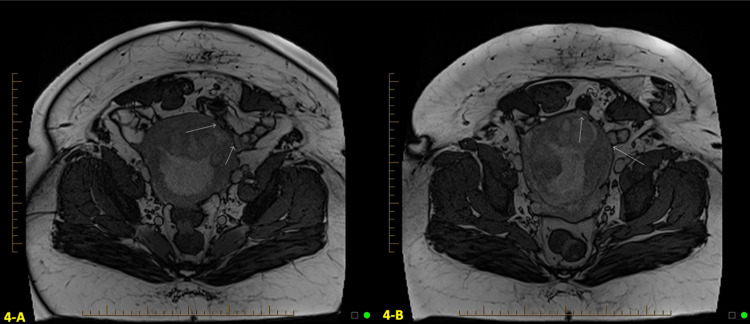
Axial fast imaging with steady-state free precession (FISP) Magnetic resonance imaging (MRI) findings at four weeks postpartum A and B: Magnetic resonance imaging (MRI) at four weeks postpartum demonstrating an anterior placenta with no evidence of gross extension of the placental tissue through the full thickness of the myometrium. The arrows point at a loop of the small bowel adjacent to the anterior left aspect of the uterine body near the area of the placental wall thickening but do not show any definitive evidence of placental soft tissue extension through the uterine wall to involve this loop of the small bowel.
